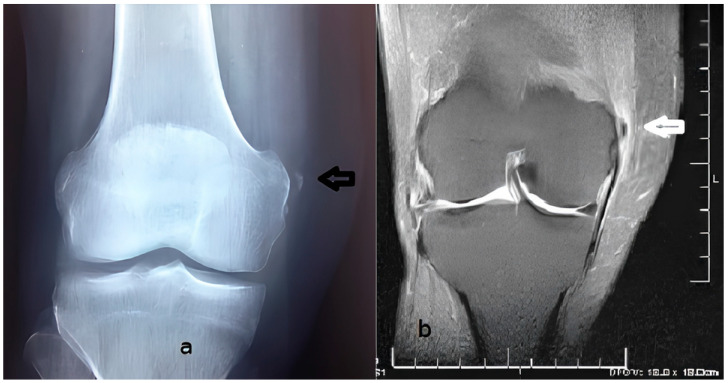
(a) Anteroposterior radiograph of the right knee showing calcification adjacent to the internal femoral condyle (black arrow). (b) Coronal PD-FS image of the right knee showing a hypointense calcification adjacent to the internal femoral condyle (white arrow).
